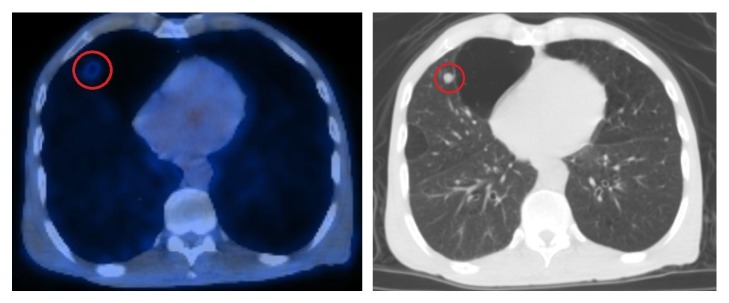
PET/CT shows a hypermetabolic spiculated lung nodule in the right middle lobe. There is background severe bullous emphysema.
